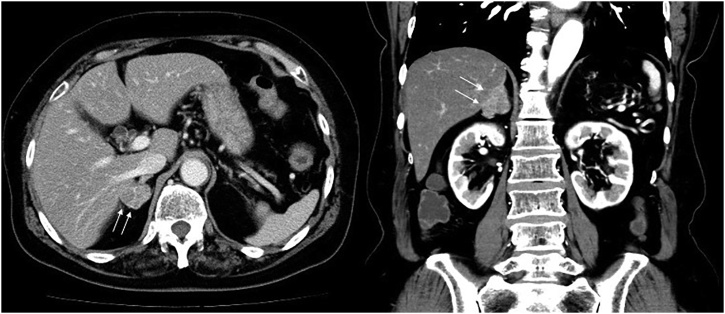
Contrast-enhanced computed tomography findings at the time of diagnosis. The images show an enlargement of the right adrenal gland that was 26 mm in size (arrows).
